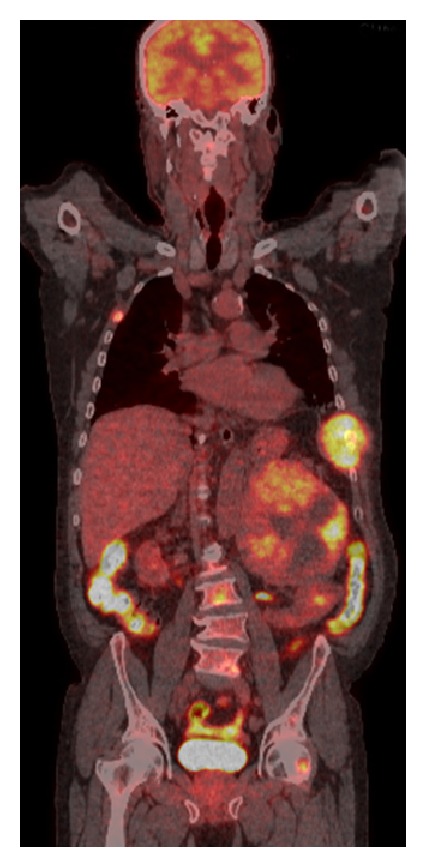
127 × 143 × 144 mm (RL × AP × KK) mass in the left adrenal with SUV max: 12.7 and widespread involvement of the skeletal system (multiple ribs, sacrum, sacroiliac area, and left femur).
